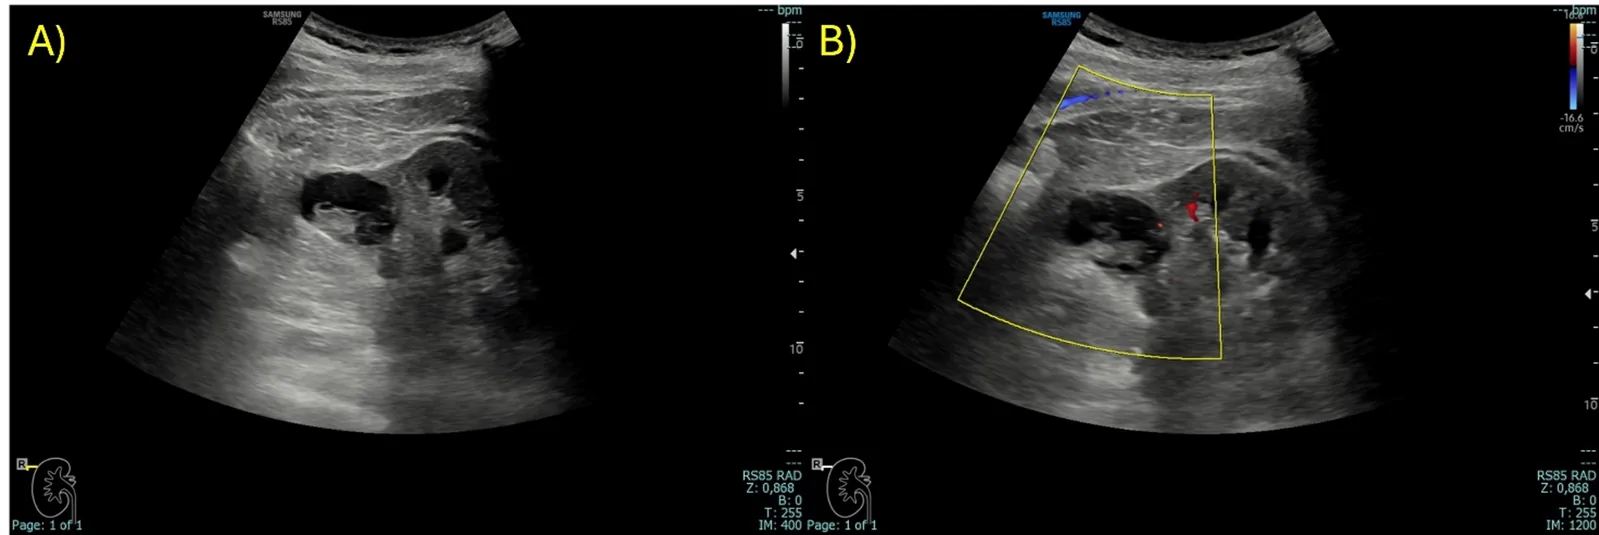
Grey-scale ultrasound images showed an exophytic, predominantly cystic renal lesion located at the right lower renal pole, with internal septa and a mural solid component (A). Colour Doppler ultrasound showed no detectable intralesional vascular signal (B), in the setting of cortical thinning and heterogeneous renal parenchymal echotexture consistent with chronic kidney disease
